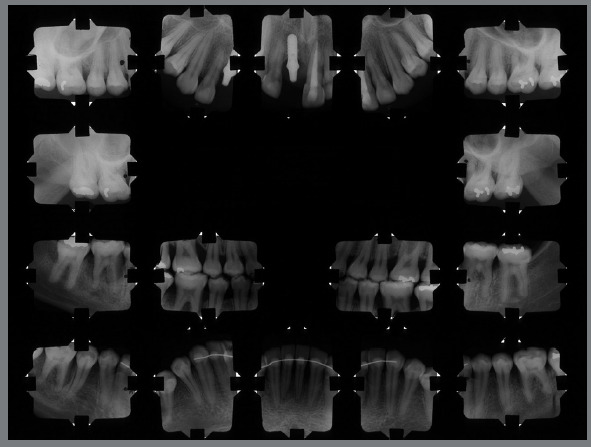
Post-treatment periapical radiographs.
